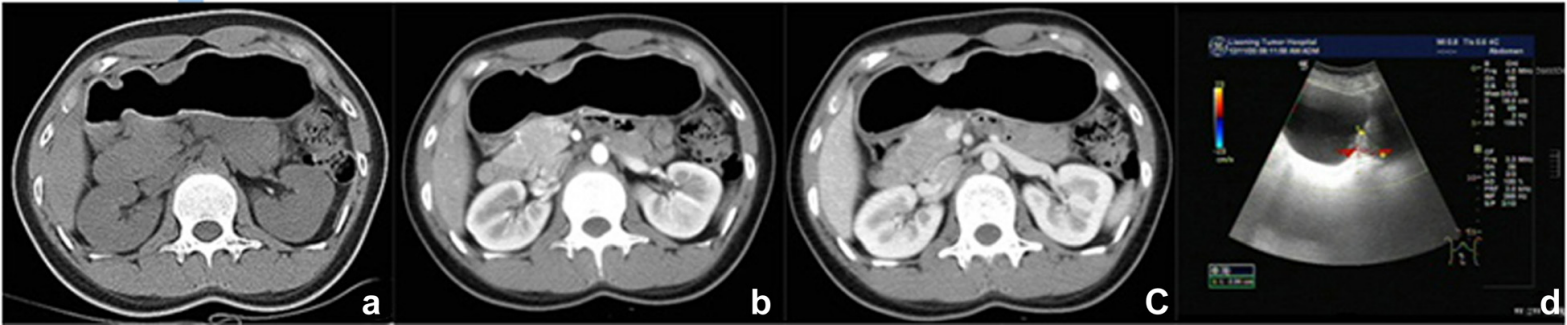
T1 tumors in lesser curvature of the gastric angle. (a-c) Enhanced CT scanning showed a clear gap surrounding the fat and smooth serosa. (d) Ultrasound imaging. Visible lesions were seen in the submucosa.
